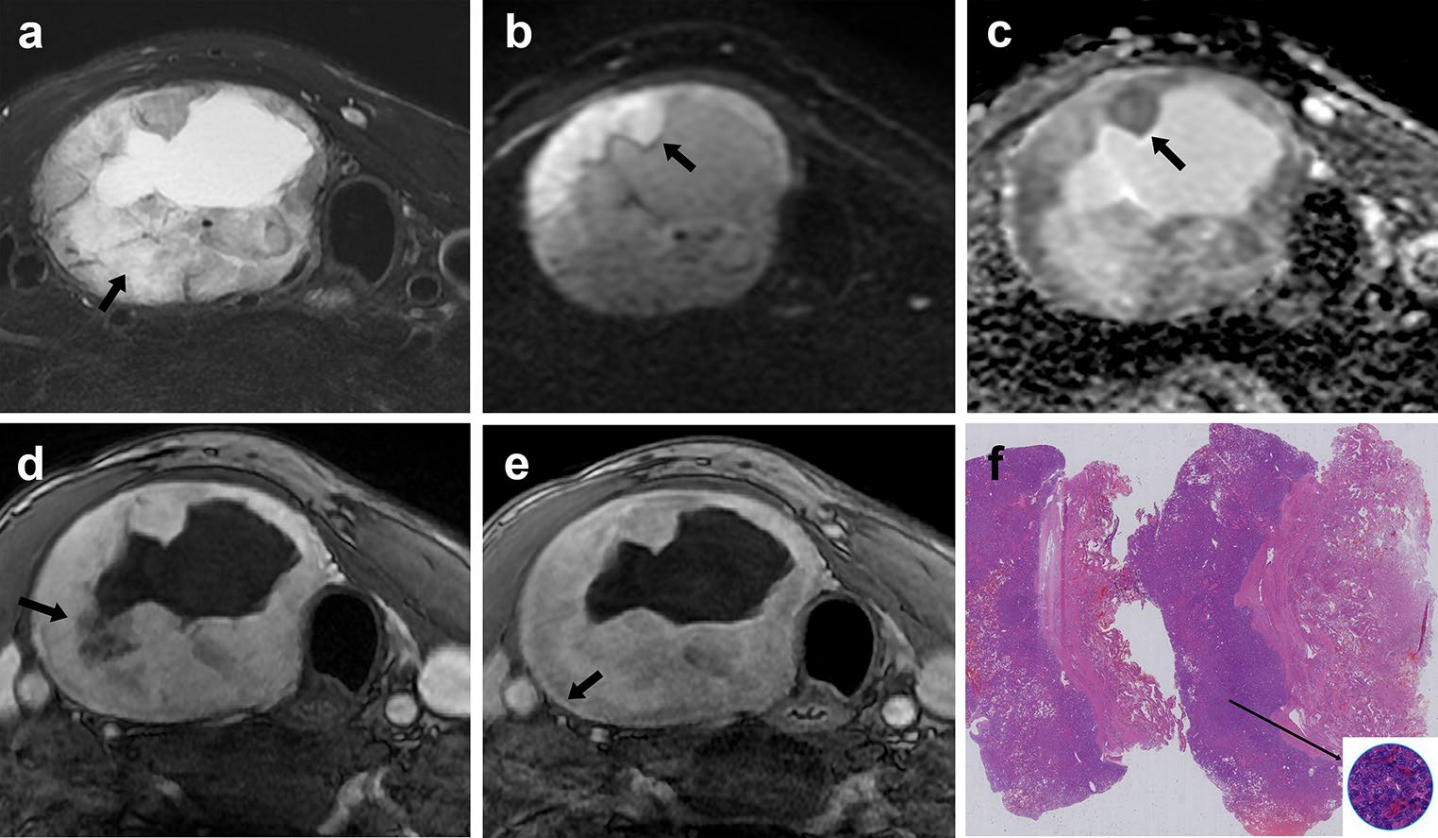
A 43-year-old man presented with a thyroid follicular carcinoma larger than 4 cm in the right lobe. Axial T2-weighted image a shows a heterogeneous mass and the present of hyperintense on T2WI with enhancement (black arrow). Axial DWI b and ADC c images show an area with restricted diffusion within the lesion (high signal on DWI and low signal on ADC, black arrow). Axial contrast-enhanced MRI (early phase) d shows a hyperintense enhancing mass and irregular fissure that did not enhance (black arrow). Axial contrast-enhanced MRI (delay phase) e shows a relatively homogeneous enhanced lesion with pseudocapsule (black arrow); fissure that do not enhanced in the early phase enhanced gradually, and the area of cystic degeneration is seen within the lesion. Pathological section analysis (HE, ×1) f shows densely distributed small follicles and follicular cells (long black arrow, HE, ×40)
